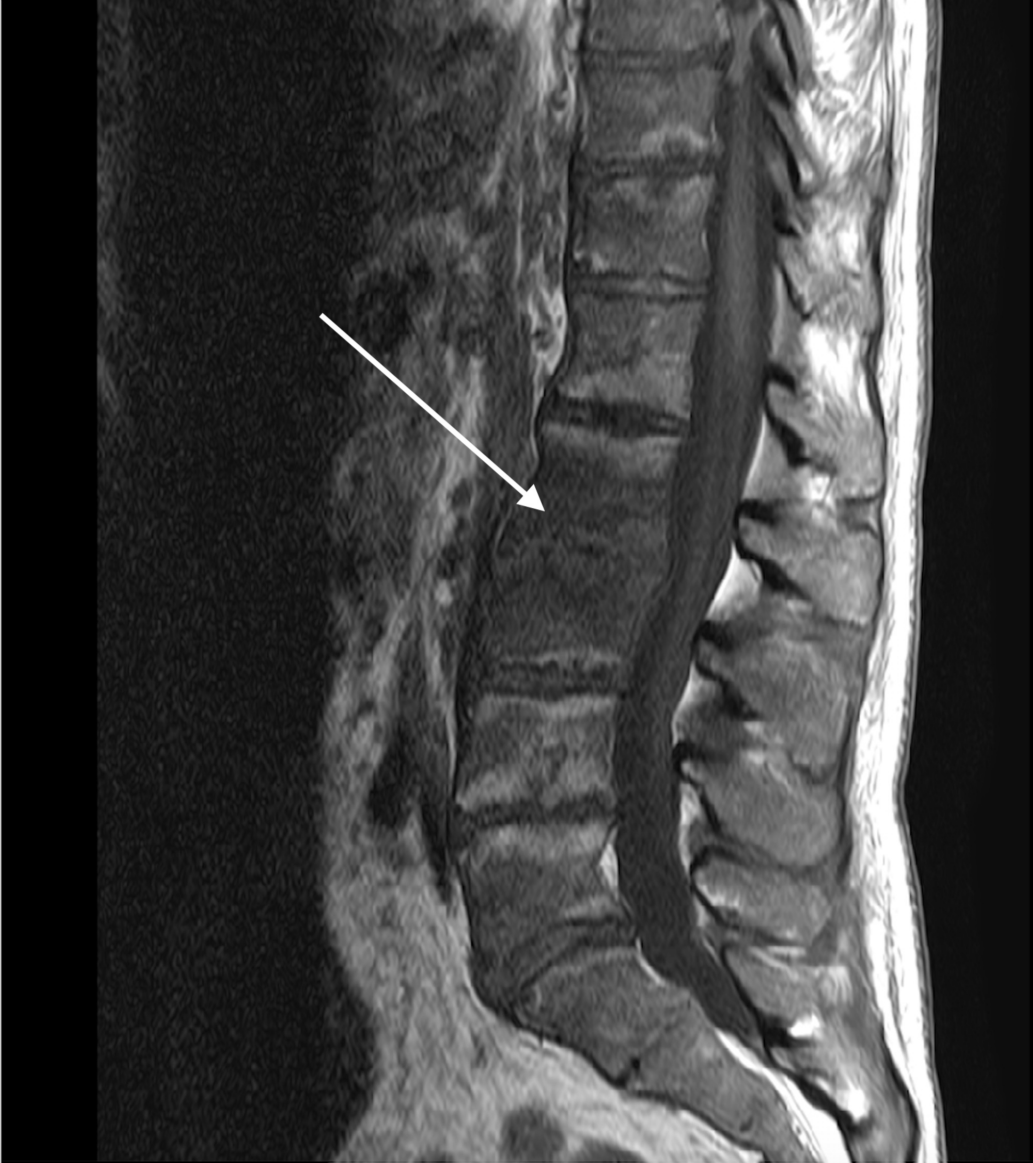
Magnetic Resonance Imaging Without Contrast of the Lumbar Spine Sagittal image revealing improvement of the acute discitis at the L2-L3 vertebral joint
